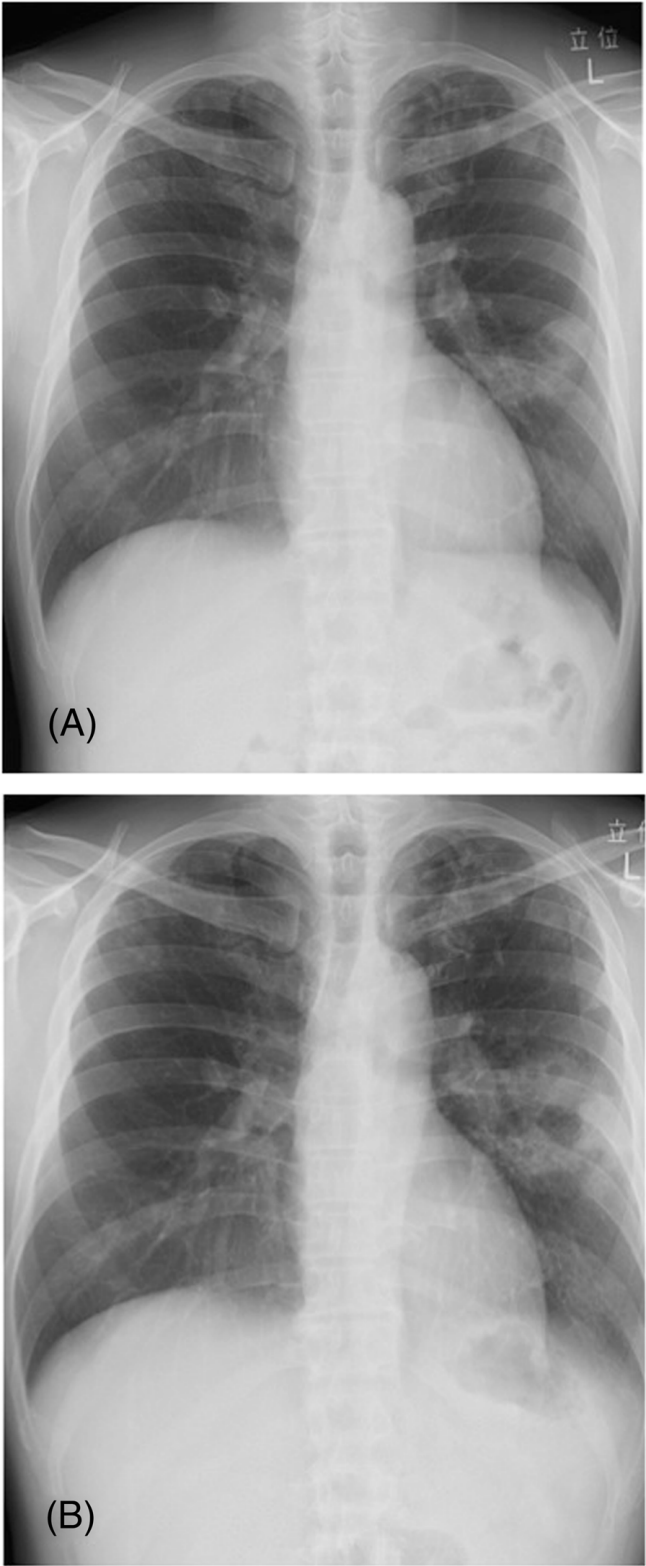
Chest X-ray in February and May 2007. Chest X-ray in February 2007 showed that the cavitary lesion in the left lung seemed stable (A). However, in May, the lesion enlarged further (B).
